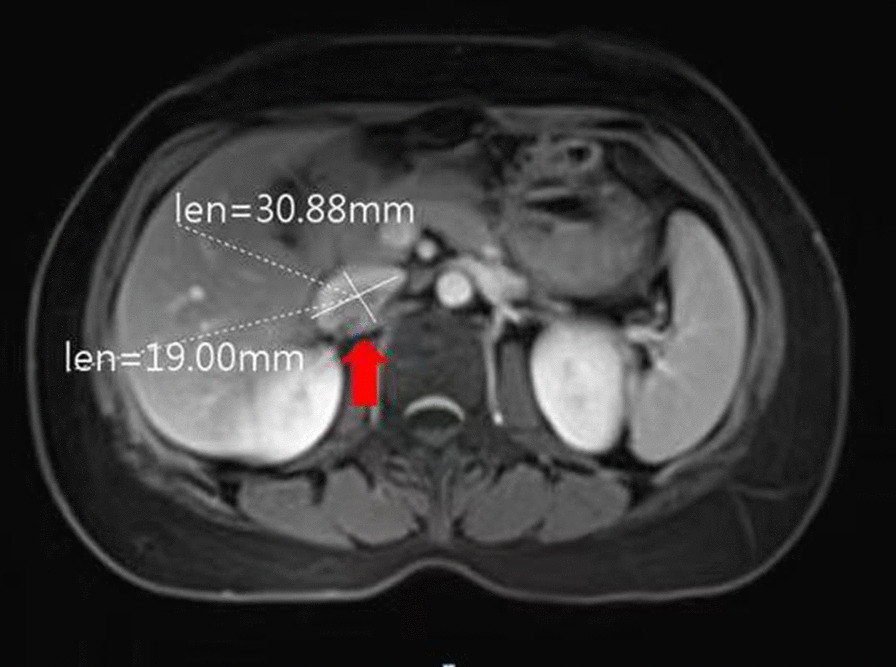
CT image of lymph node metastasis in the abdominal aorta
